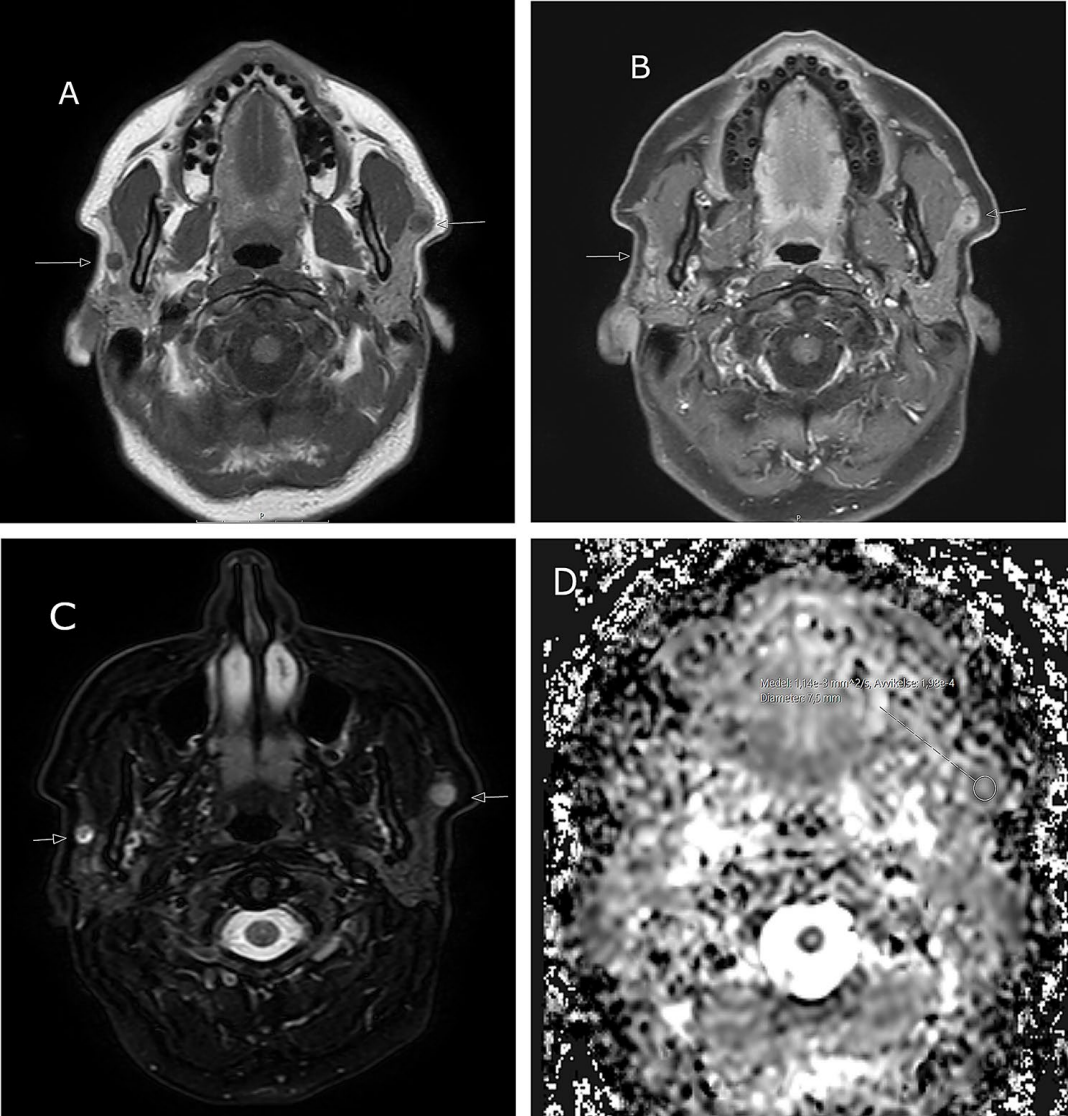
Patient 2, MR image of bilateral salivary gland tumours in a 50-year old female. A-D Demonstration of bilateral intraparotid lesions in the superficial portions. A) Low signal on T1W (arrows), B) rich, irregular contrast enhancement on fat-suppressed TIW (arrows), C) high signal on fat-suppressed T2W (arrows) and D) restricted diffusion of the left sided lesion on the ADC-map, ADC = 1,14 × 10^− 3^ mm^2^/s
